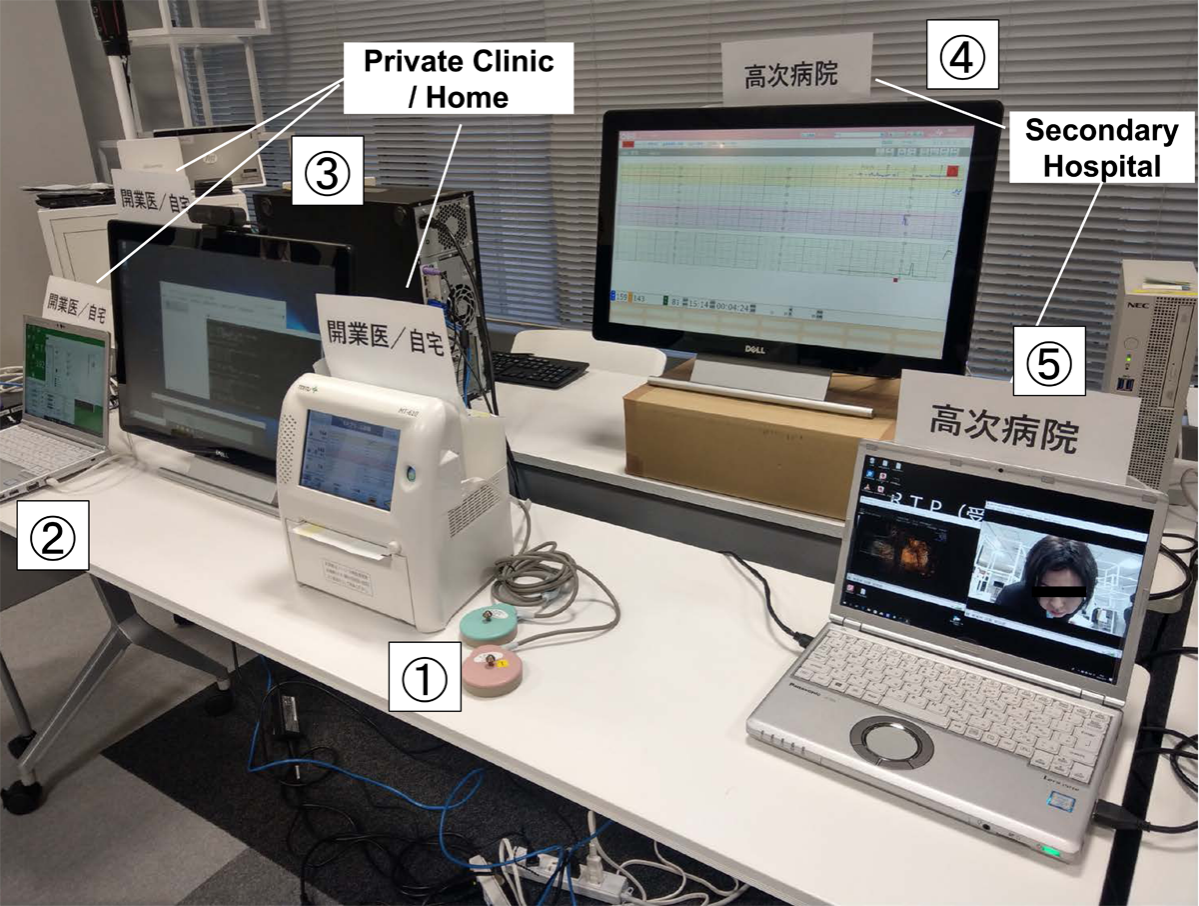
Panoramic view of the experiment. Objects 1-3 are the source side, and objects 4 and 5 are the receiving side of the 5G transmission system. 1: The cardiotocogram (CTG) device (MT-610) transmits the waveform of the fetal heart rate in clinical practice; 2: the computer plays back ultrasound examination videos; 3: the computer plays back movies from a video camera (operation of the real-time self-taken video is checked afterwards); 4: the CTG waveform display is maximized at the receiving end to check visibility; 5: movies of the ultrasound examination (left) and video camera (right) are played simultaneously at the receiving end.
